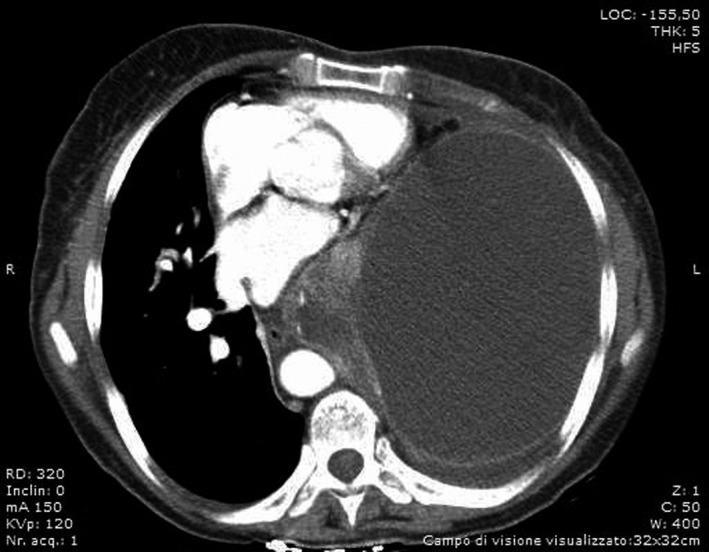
CT scan shows mixed high‐density pleural fluid accumulation in the left and atelectasis of the lung
